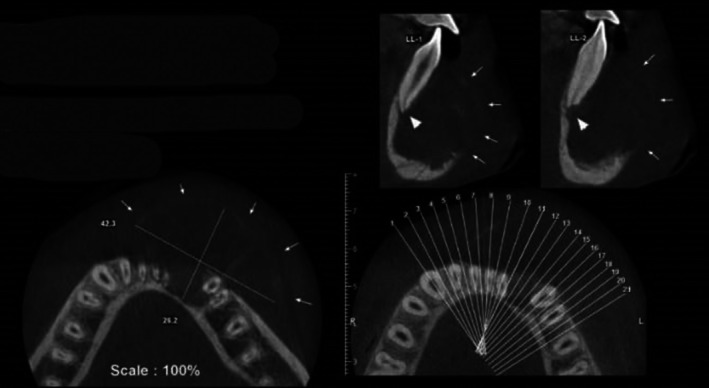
Axial and sagittal CBCT image demonstrating a well‐defined expansile lesion with perforation of the buccal cortex.
